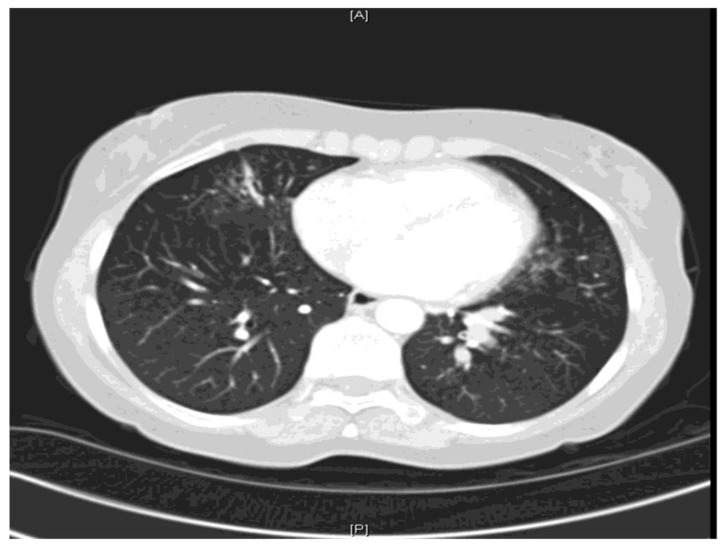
CT thorax showing extensive mucus plugging within the left lower lobe.
